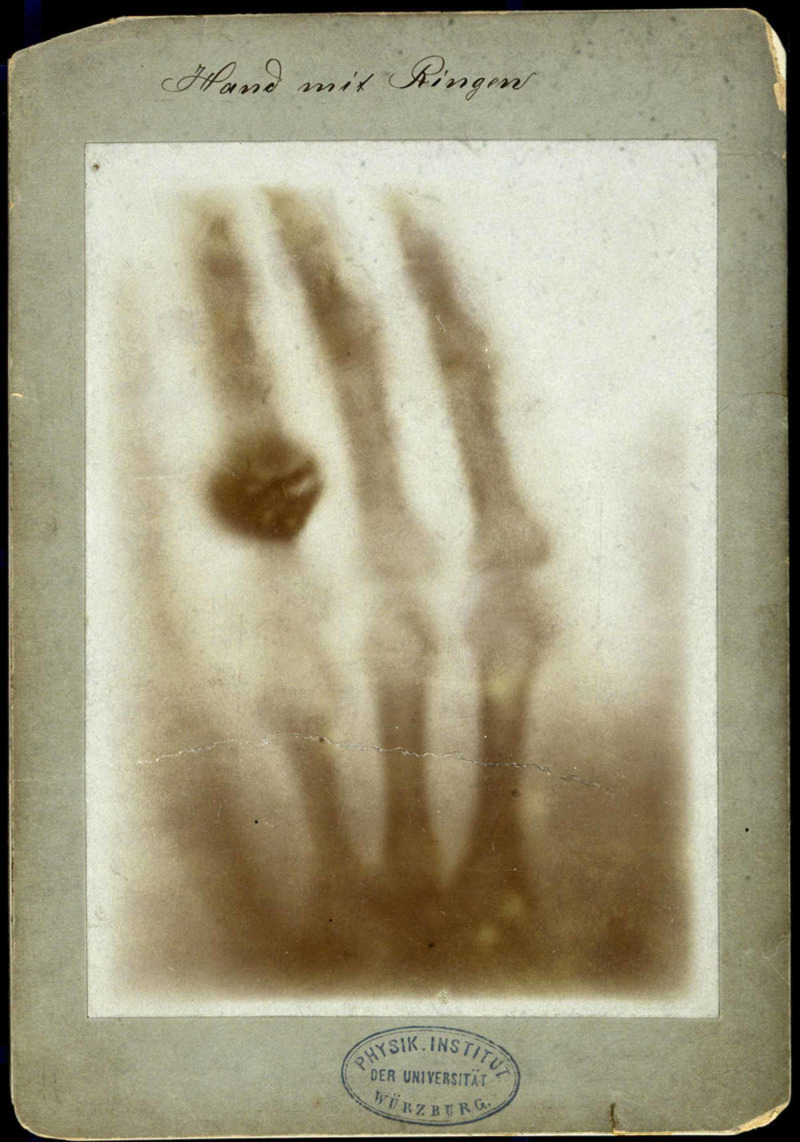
The first x-ray image, “Hand mit Ringen” Courtesy: https://www.atlasobscura.com/articles/roentgen-xrays-discovery-radiographs
